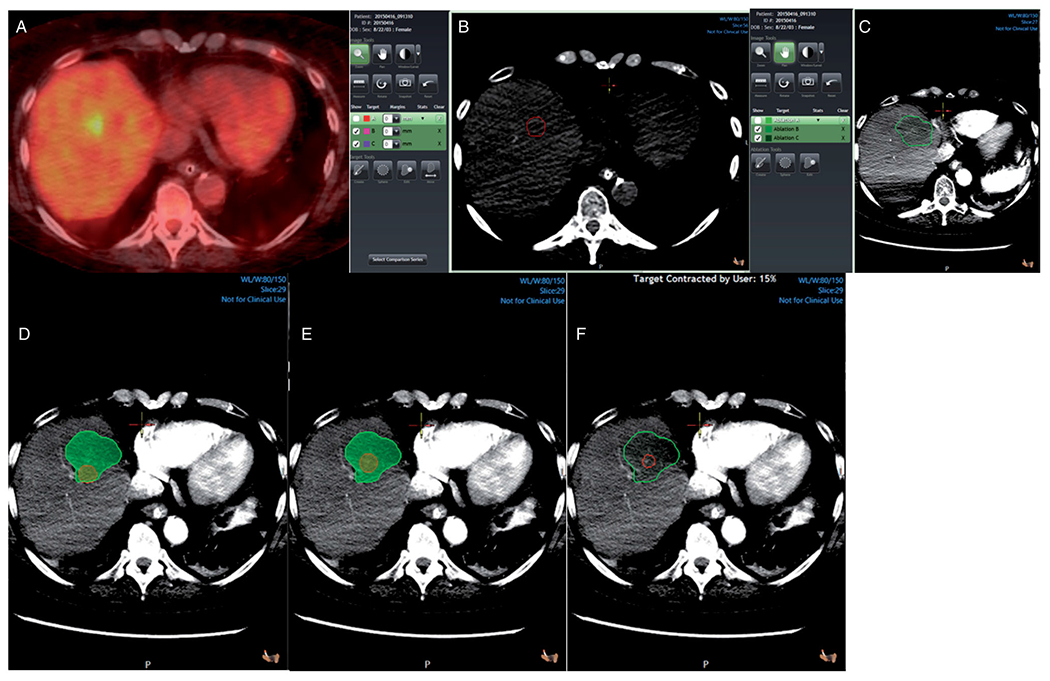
3 D margin assessment in a patient with colorectal liver metastasis in segment 8. Pre-ablation PET/CT scan showing the tumor (A). Tumor segmentation (red) in a pre-ablation CT scan (B). Ablation zone segmentation (green) in a post-ablation contrast-enhanced CT scan (C). Tumor (red) and ablation zone (green) registration without target movement showing ablation margins <5mm (D). Tumor (red) and ablation zone (green) registration with target movement to match anatomic landmarks (E). Tumor (red) and ablation zone (green) registration with target movement and tissue contraction algorithm applied (F).
